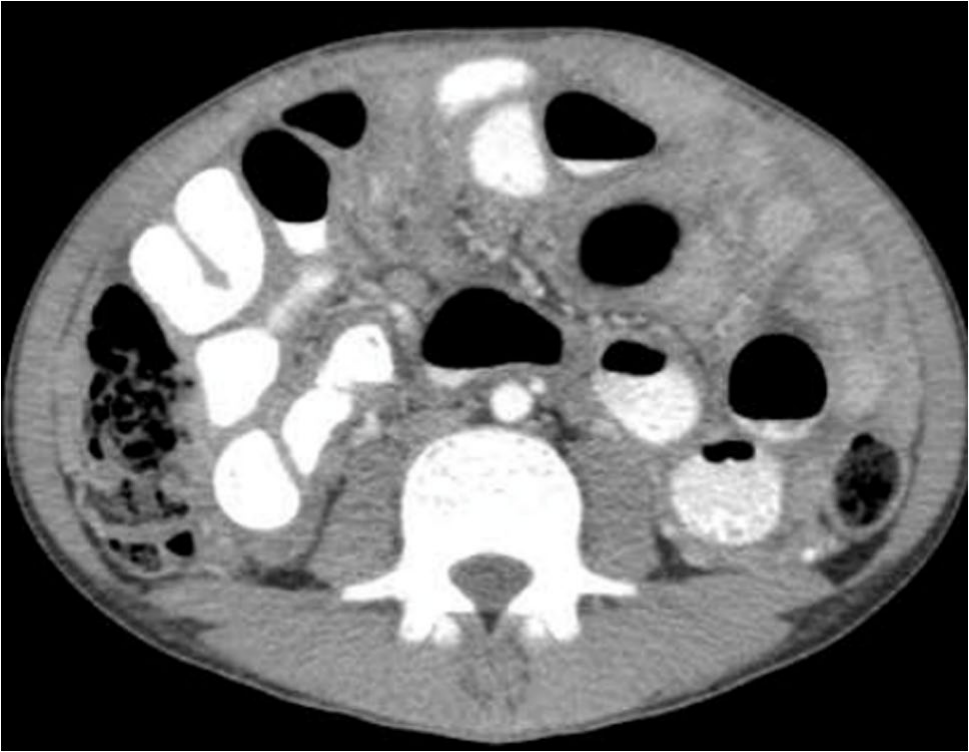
An axial computed tomography image of the mid-abdomen following intravenous and oral contrast in a 3-year-and-5-month-old boy shows diffuse thick hazy stranding of the peritoneum separating the intestinal loops, in keeping with peritoneal tuberculosis of the dry plastic type
